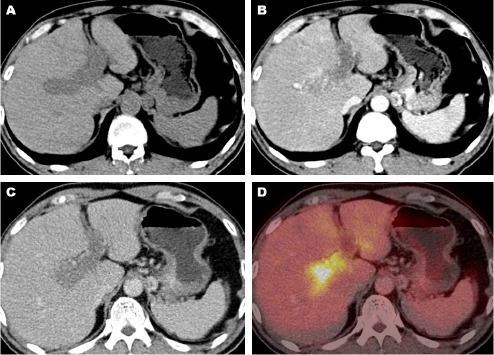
The contrast-enhanced FDG PET/CT image shows the expansion of right and left portal veins with hypodense intraluminal thrombus in non-enhanced CT scan (A). The thrombus in the right branch showed a linear contrast enhancement in the arterial phase CT (B). The thrombus appeared washout in the portal venous phase CT (C), and showed an increased FDG uptake in the PET/CT image (D)
